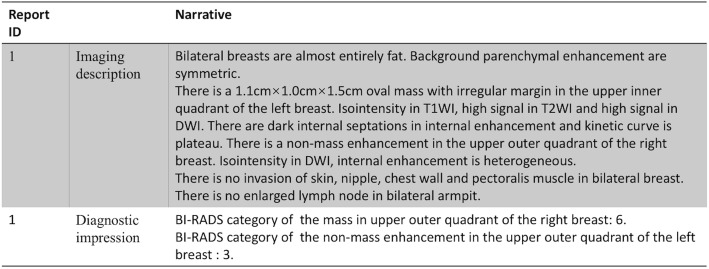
A representative original breast MRI report. The report consists of an imaging description and diagnostic impression
